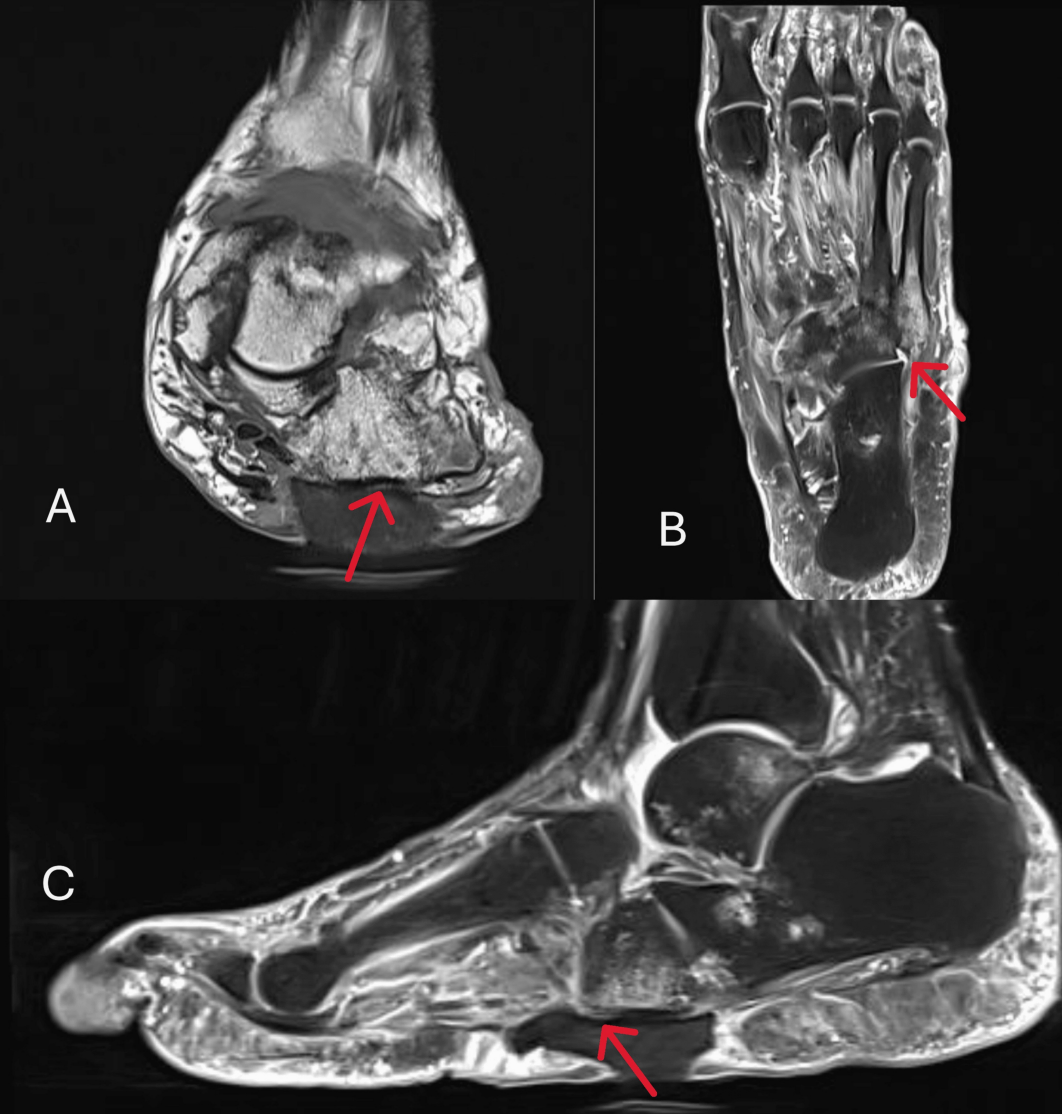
MRI of the left foot — (A) axial, (B) coronal, and (C) sagittal views — demonstrating a gas-containing soft tissue defect at the plantar lateral aspect of the midfoot, with the deep margin of the defect bordering the cuboid bone, and pronounced marrow edema in the cuboid and fifth metatarsal base with corresponding low T1 signal intensity and loss of cortical definition, consistent with osteomyelitis The arrows indicate the affected cuboid and base of the fifth metatarsal, showing marrow edema and cortical erosion in all three planes.
